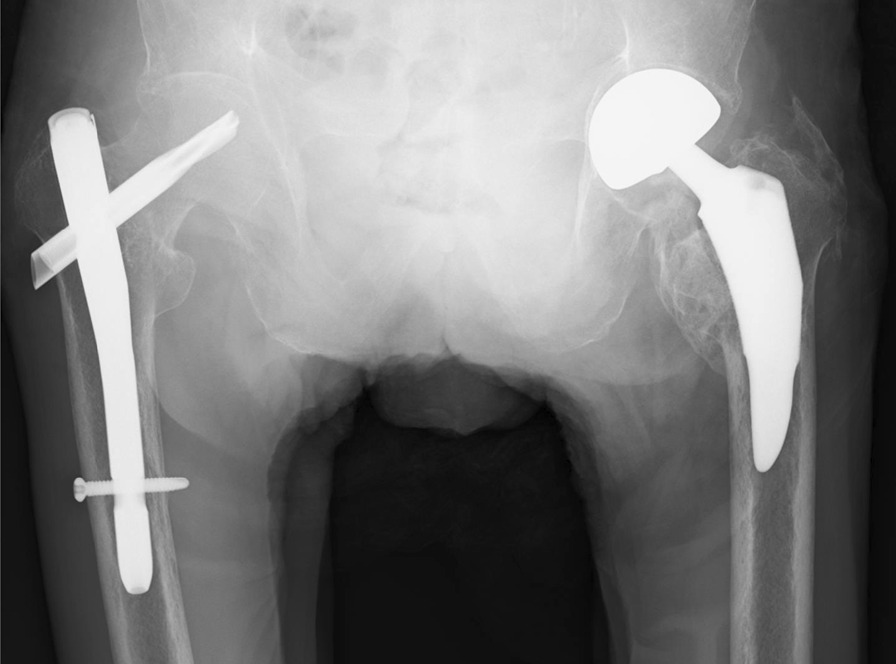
Radiography findings 13 months after the operation. Radiograph revealed absence of loosening of the left hemiarthroplasty and heterotopic ossification of the left hip joint, and bone fusion was obtained in the right trochanteric fracture
